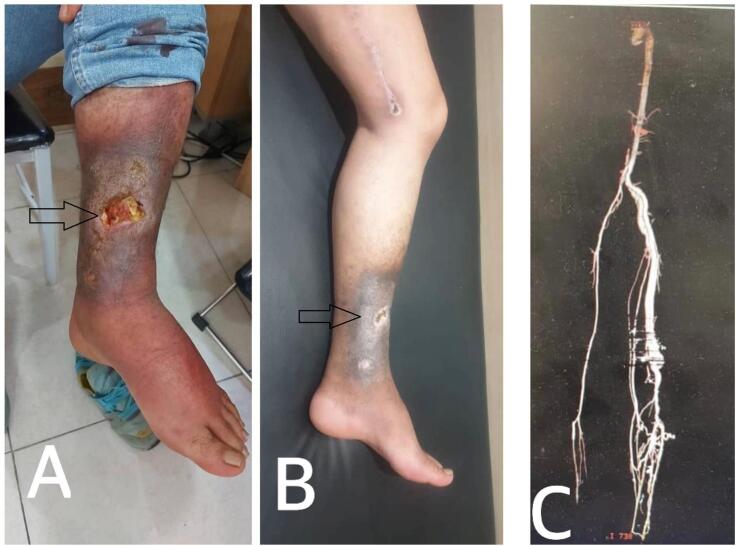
A: Left leg before surgery; B: Left leg after surgery; C: CT angiography of the left lower extremity.
